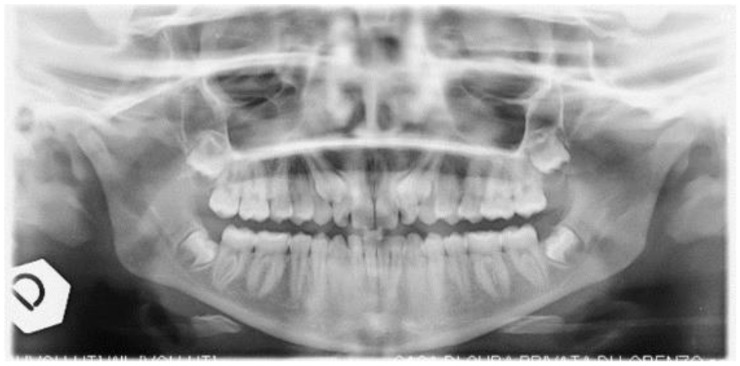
Orthopanoramic X-ray pre-treatment.
